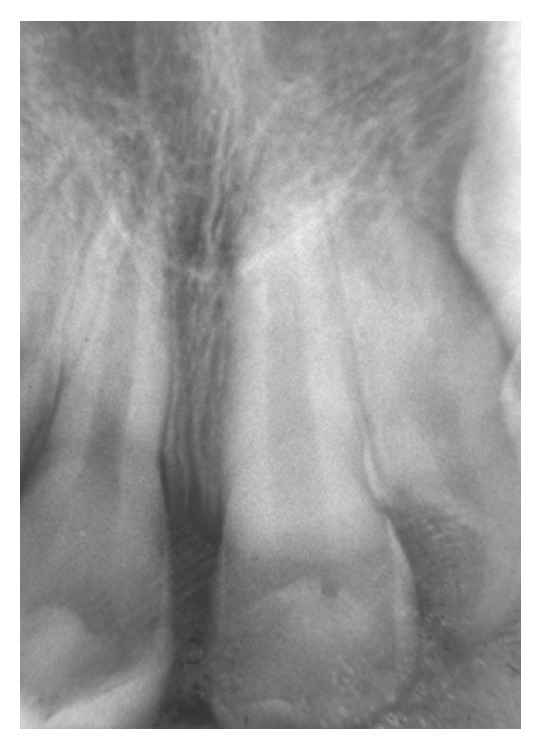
The initial periapical radiograph revealed the absence of fracture lines on the root structures, increased periodontal ligament spaces apically, or any radiolucent lesion in apical area of the injured incisors (first session; March 1998).
